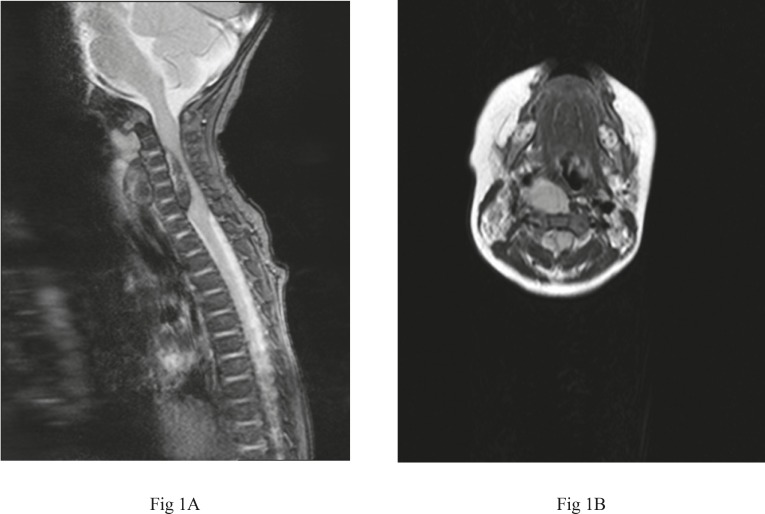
MRI of the spine showing an epidural hematoma extending from C4 to C7 leading to displacement and compression of the spinal cord with expansion of segments proximal and distal to the site as well as a hematoma in pre and paravertebral spaces on axial view. A Sagittal T2-weighted image (TR 550 ms, TE 24 ms); B Axial T2-weighted image
